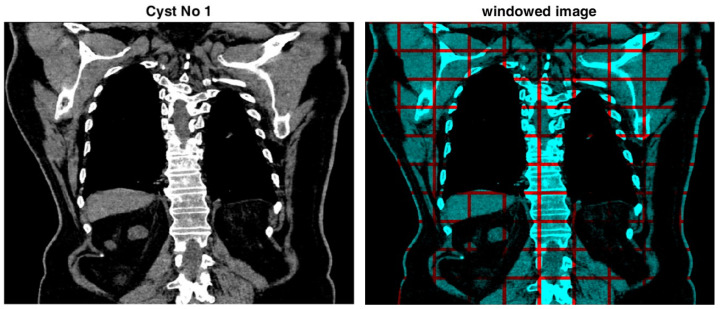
An original CT image (on the left) and the corresponding windowed image (on the right).
